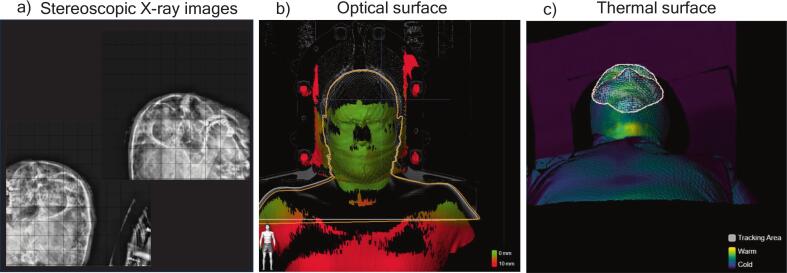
Visualisation of the different system features. A: a pair of stereoscopic X-ray images of a skull. The images are taken from each side of the treatment couch in oblique angles. B: optical surface (green/red). C: thermal information (gradients purple to green) and a marked ROI used for surface scanning (white). (For interpretation of the references to colour in this figure legend, the reader is referred to the web version of this article.)
